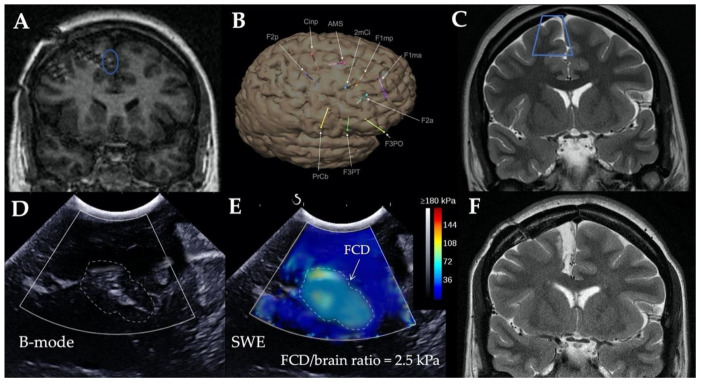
Preoperative MRI images ((A) T1- and (C) T2-weighted, coronal views), in a patient with right mesiofrontal cryptogenic epilepsy. Intracranial electroencephalogram (EEG) recordings located epileptogenic zone around deepest contact of the F1mp electrode ((A), blue circle, and (B)). (C) Blue trapezium: ultrasound imaging plan. (D) Intraoperative ultrasound B-mode images did not find any abnormality, while (E) elastography images detected small heterogeneous area of stiffer parenchyma consistent with focal cortical dysplasia. (F) Right medial frontal surgical resection allowed for patient to achieve seizure freedom. FCD, focal cortical dysplasia; SWE, shear wave elastography.
